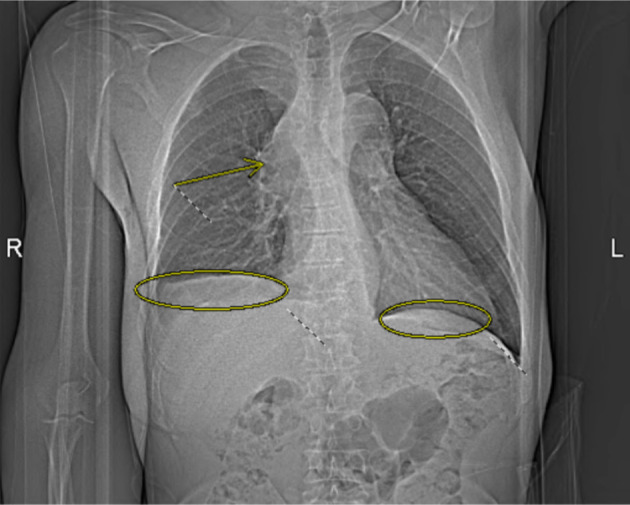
Computed tomography angiography (CTA) pulmonary chest X-ray obtained on the day of admission demonstrates bilateral pleural effusions (circled) and a mediastinal mass causing severe superior vena cava (SVC) compression and right upper lobe pulmonary artery obstruction (arrow).
